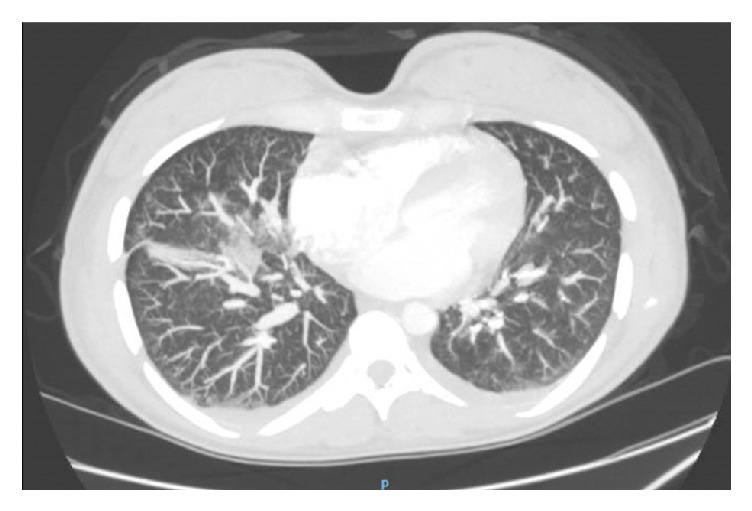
Chest computed tomography angiography (CTA) on the day of admission noting patchy infiltrate in the right middle and lower lung lobes and small bilateral pleural effusions.
